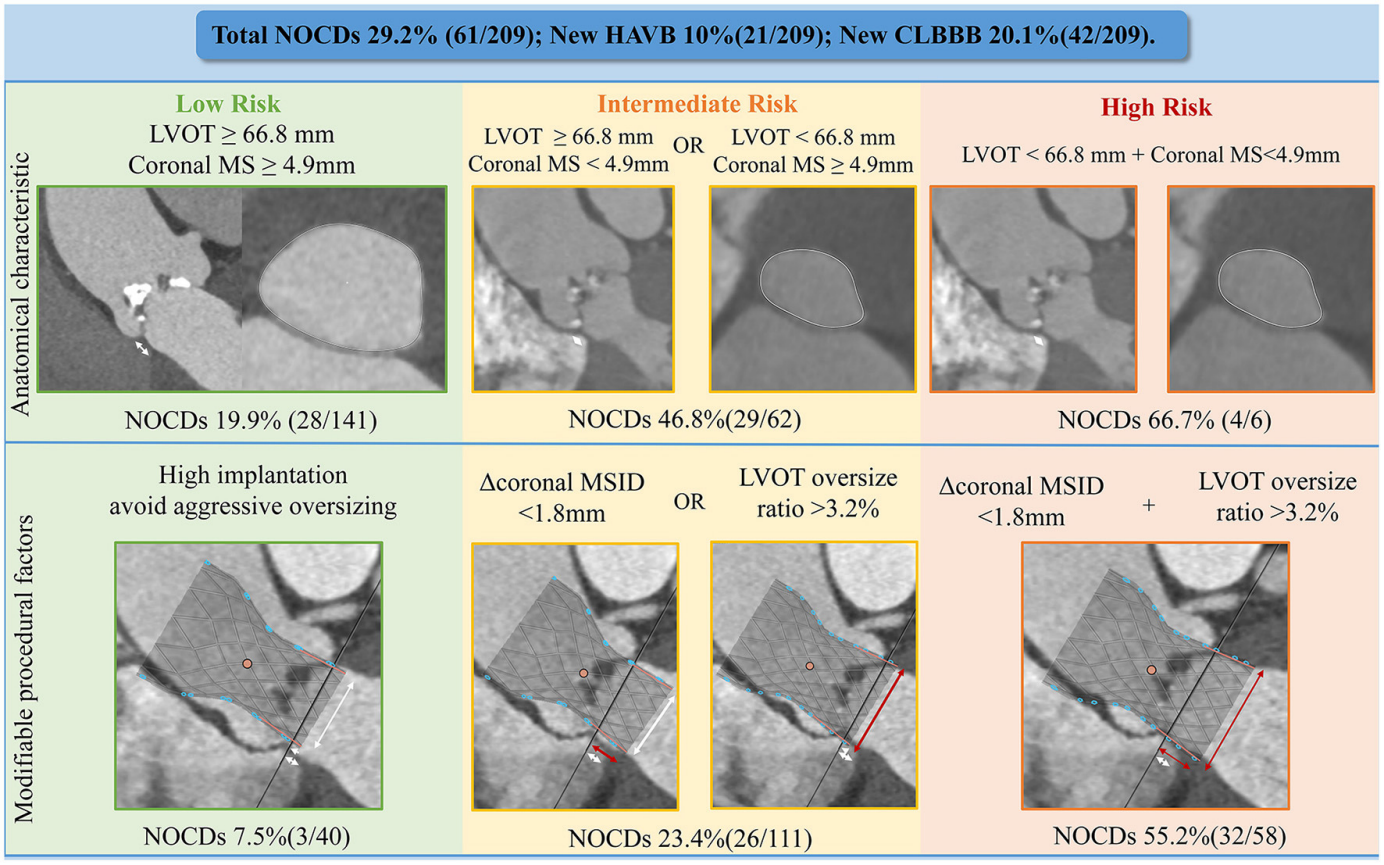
Predictive model of NOCDs. NOCDs, new-onset conduction disturbances; HAVB, high-grade atrioventricular block; CLBBB, complete left bundle branch block; LVOT, left ventricular outflow tract; Δcoronal MSID, coronal MS length minus implantation depth on CT.
